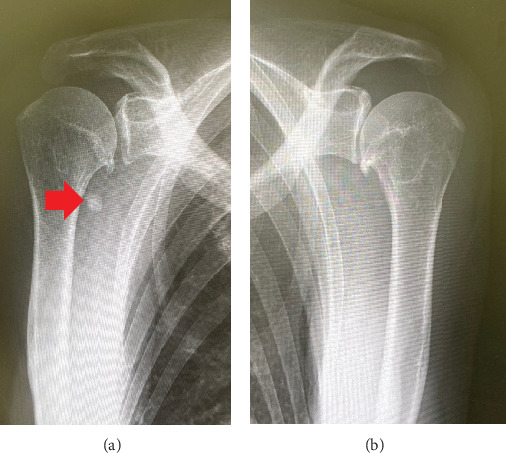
Plain radiographs of the bilateral shoulder joints. (a) Right knee and (b) left knee. (a, b) Anteroposterior view. Synovial osteochondromatosis was confirmed on the right side.
